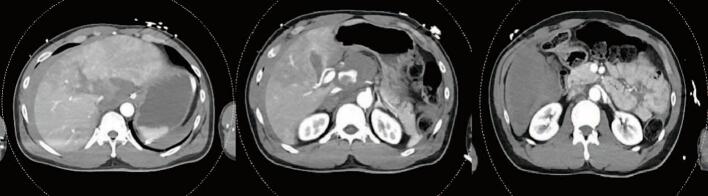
Admission CT Abdomen/Pelvis CT Abdomen/Pelvis showing a AAST Grade V liver injury with extension to the IVC and subhepatic hematoma. Additional bleeding from a branch of the right hepatic artery was seen in the right hepatic lobe.
